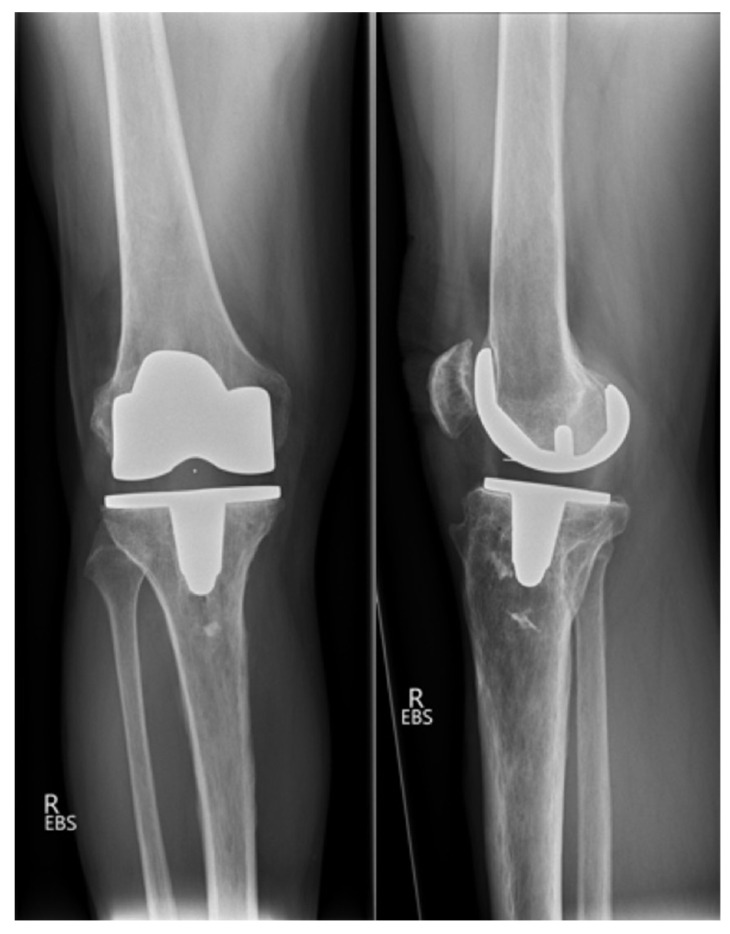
Anteroposterior and lateral non-weight bearing radiographs of the right knee after primary posterior cruciate retaining TKA.
